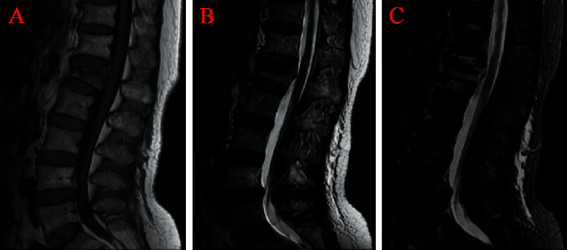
Posterior fascia oedema of osteoporotic vertebral compression fractures was visible on (a) T1 WI, (b) T2 WI, and (c) T2-STIR WI of MRI.
